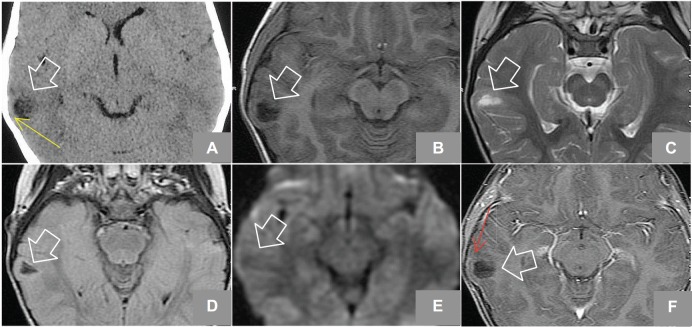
(A) Axial Non enhanced CT shows hypodense lesion in right temporal region, lobulated that shapes the adjacent bone tissue (yellow arrow). MRI axial sequences of: (B) T1w, (C) T2w, (D) FLAIR (Fluid-Attenuated Inversion Recovery), (E) diffusion weighted, and (F) T1w + gadolinium which identifies an image (white arrows) hyperintense on T2w, hypointense on T1w, and FLAIR and does not restrict diffusion w; it is located at the level of the second right temporal gyrus in its portion with discrete anterior subcortical gliosis and lateral enhancement after gadolinium administration (red arrow).
